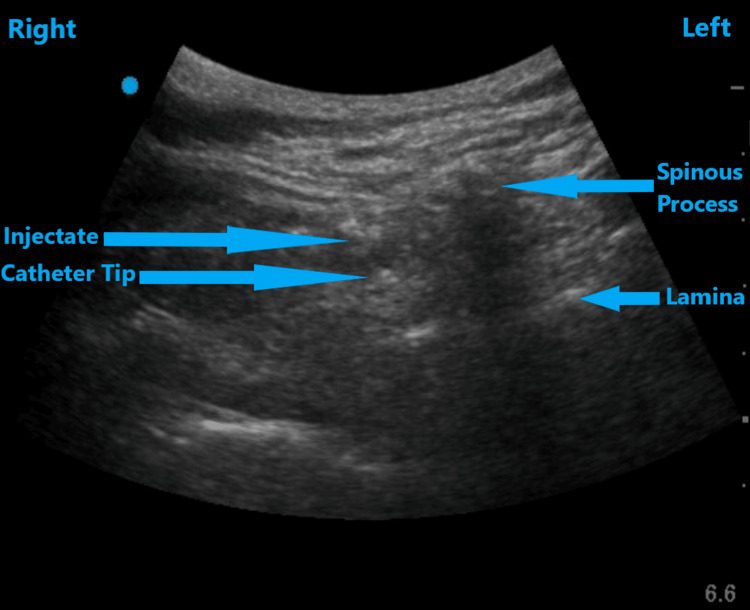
Ultrasound image of the thoracic spine in transverse view, demonstrating the catheter outside the epidural space
